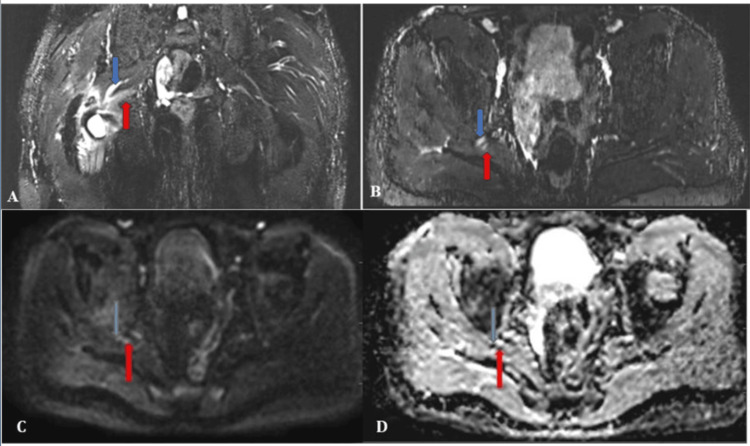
A) Coronal MIP image; B) Axial MIP image; C) DWI; D) ADC MIP: maximum intensity projection image; DWI: diffusion-weighted imaging; ADC: apparent diffusion coefficient Hyperintensity and increased thickness are noted in the right sciatic nerve (shown by blue arrow) and right posterior cutaneous nerve (shown by red arrow) Figures (C) and (D) show diffusion restriction with a low ADC value
